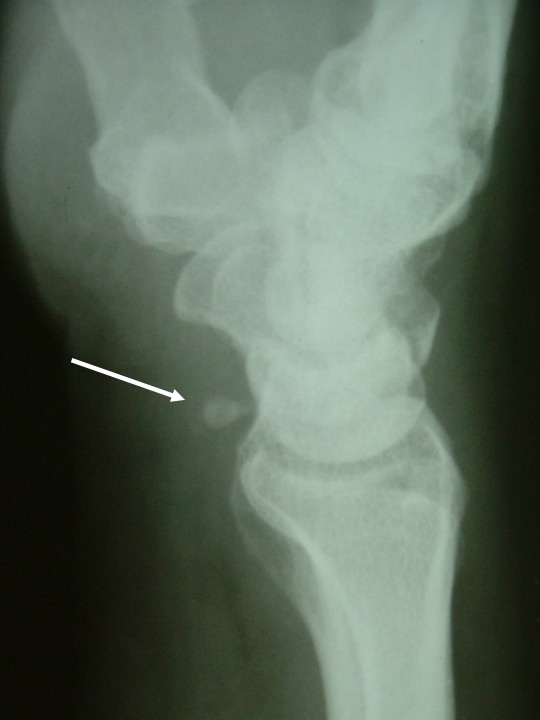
Lateral radiograph of the wrist showing the sesamoid anterior to the triquetrum.
